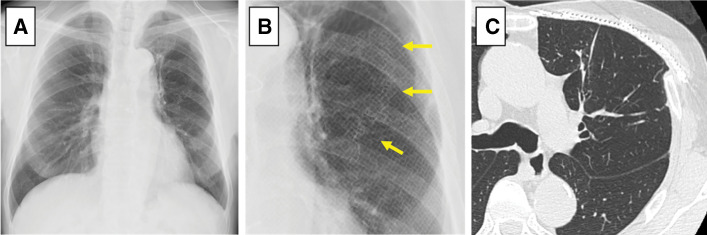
Radiological findings 3 years after surgery. (A) Chest radiograph showing good lung inflation. (B) Zoomed chest radiograph showing a crack in the titanium mesh plate (yellow arrows). (C) CT showing no significant lung damage, and that the reconstructed material is well-aligned.
